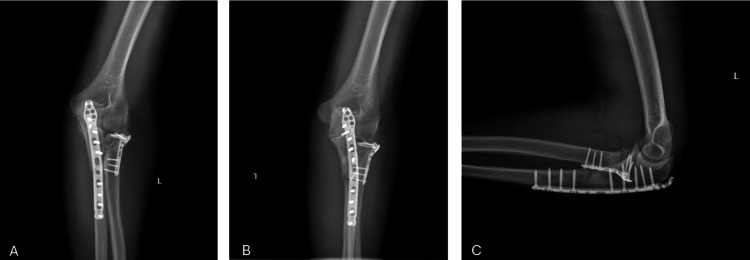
The patient's ninth-month follow-up radiographs: (A) anteroposterior (AP) view, (B) oblique view, and (C) lateral view are shown
